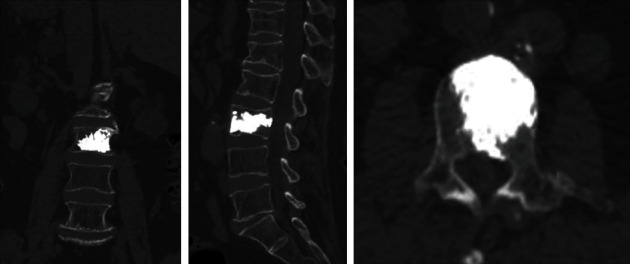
The patients were followed up for 1 year after operation with coronal, sagittal, and axial CT and the bone cement was fully diffused.
